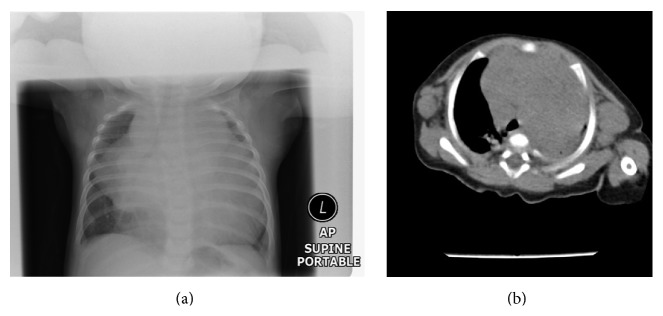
X-ray and CT scan of chest on presentation showing a large mediastinal mass with axillary lymphadeonpathy.
